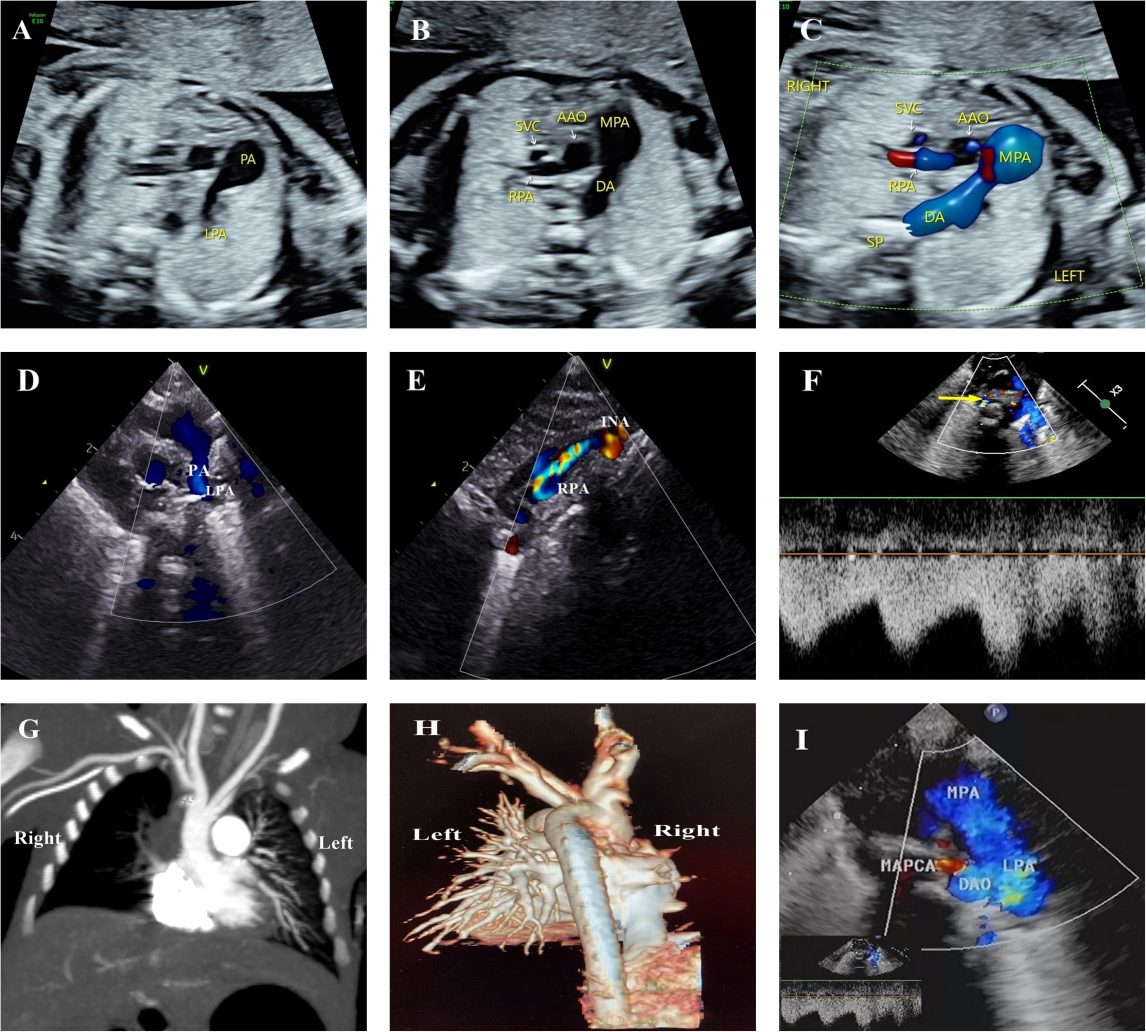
Progressive atresia of the right pulmonary artery originating from the ascending aorta in one of twins. (A–C) Main pulmonary artery continued only as the left pulmonary artery and ductus arteriosus. The right pulmonary artery originated from the ascending aorta, and a crest-like protrusion was seen at the opening of the right pulmonary artery at 24^+3^ weeks, reporting consistent results at 27^+5^ weeks and 31^+3^ weeks. (D, E) Echocardiographic review on the 1st postnatal day was consistent with prenatal results, with the right pulmonary artery originating from the lateral wall of the ascending aorta in the vicinity of the opening of the innominate artery. The course was tortuous, with the color Doppler image indicating colorful blood flow signals. (F) Cardiac ultrasound on the 25th postnatal day revealed a thickening of the main pulmonary artery and left pulmonary artery, and the small collateral vessels originating from the descending aorta (indicated with a yellow arrow). (G,H) Postnatal CTA examination and VR reconstruction only showed the left pulmonary artery branching from the main pulmonary artery, with the right pulmonary artery absent. However, no collateral vessels from the descending aorta were seen. (I) Repeated echocardiography on the 30th postnatal day did not expose the right pulmonary artery but revealed thicker collateral blood vessels from the aortic arch and descending aorta. MAPCA, major aortopulmonary collaterals.
